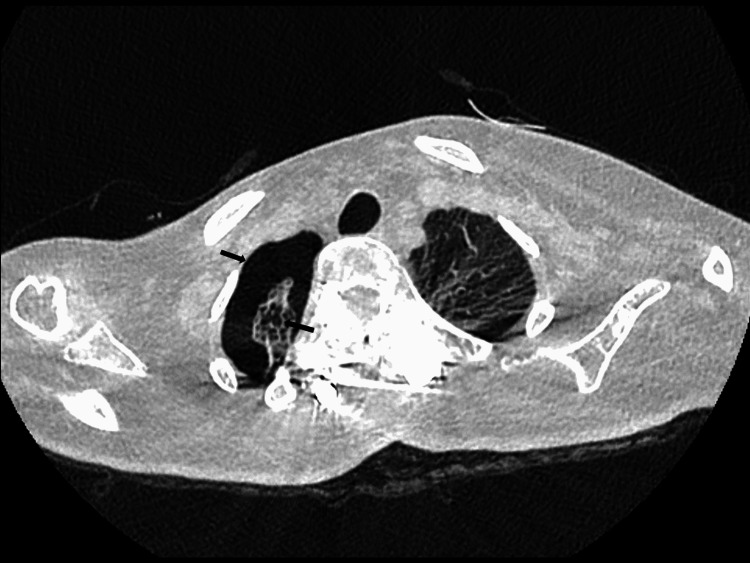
Axial computed tomography image shows multiple sub-centimeter cysts vs blebs in the right upper lobe parenchyma with pneumothorax (black arrows).
